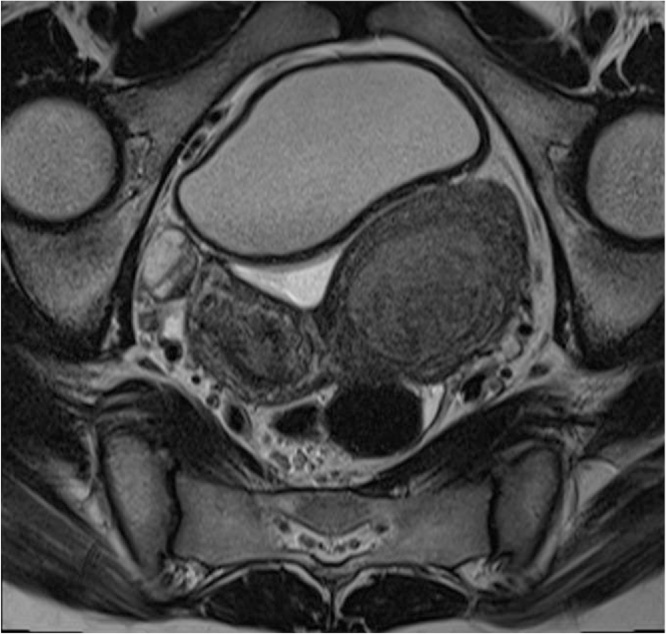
Pelvic MRI, transverse T2-weighted image, depicting a duplicated uterus and the presence of two endometrial cavities: right-sided cavity with 3.8 mm thickness and distended left cavity filled with hyperintense material.
